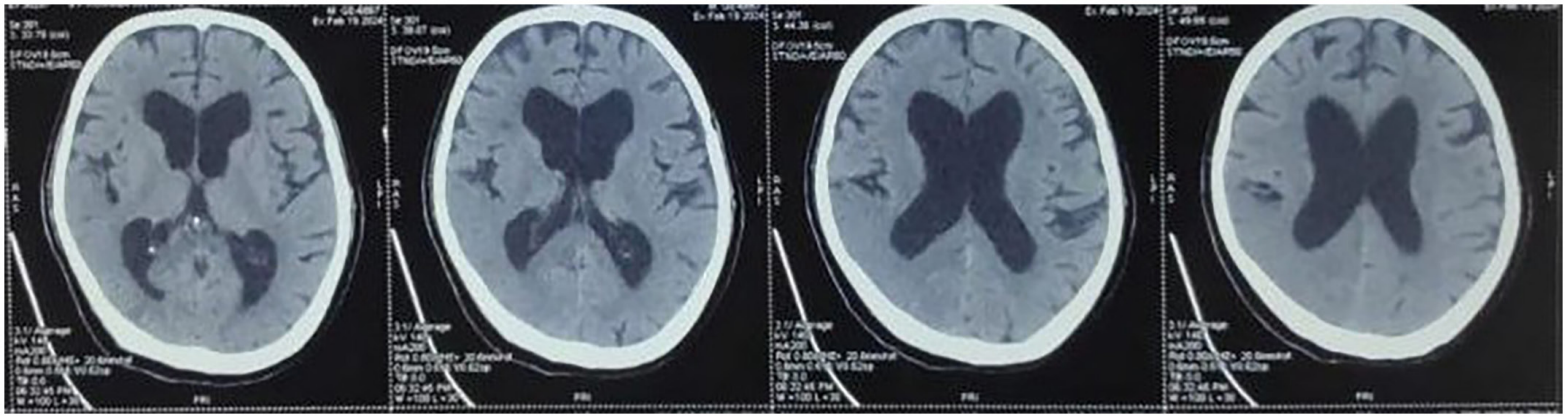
The CT head showing dilated lateral and third ventricle with mildly prominent bilateral sylvian fissures, cortical sulci, ventricular system, basal cisterns, and cerebellar folia suggestive of diffuse brain atrophy.
